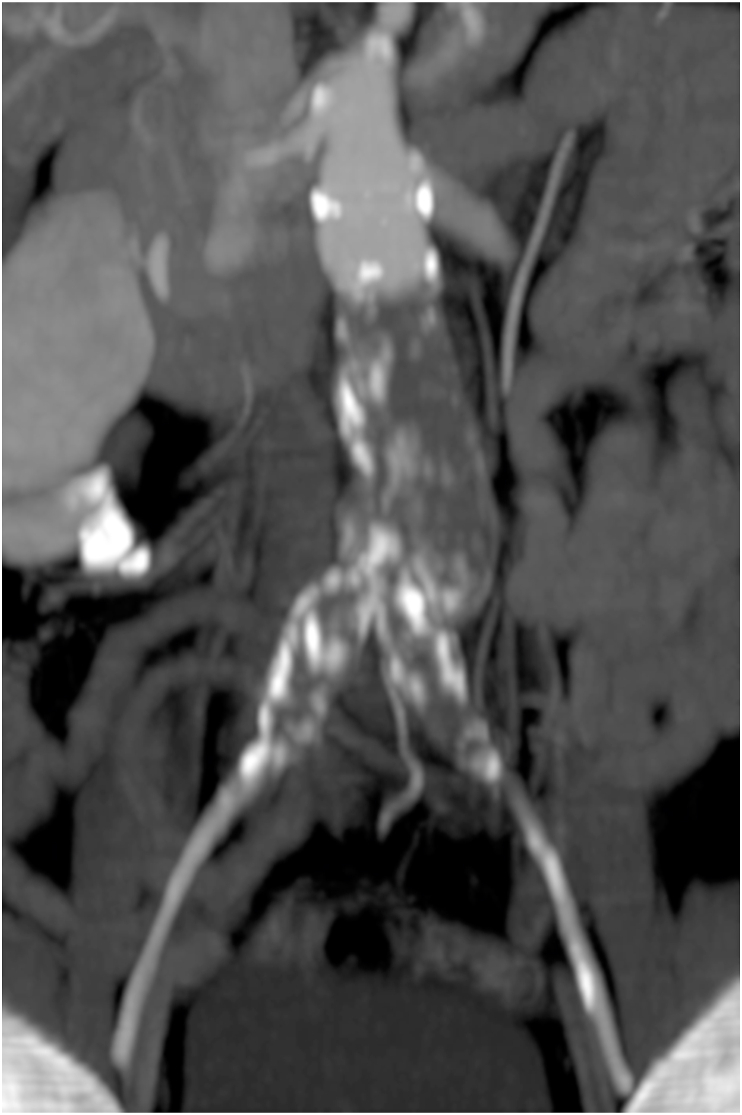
Computed tomography reconstruction of graft thrombosis.
